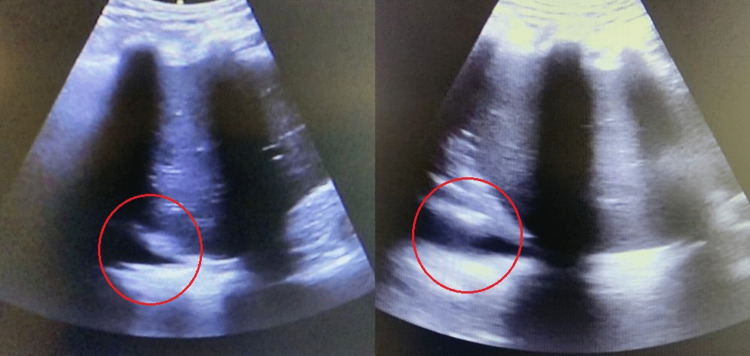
Bedside ultrasound Red circles highlight subdiaphragmatic pleural effusion
